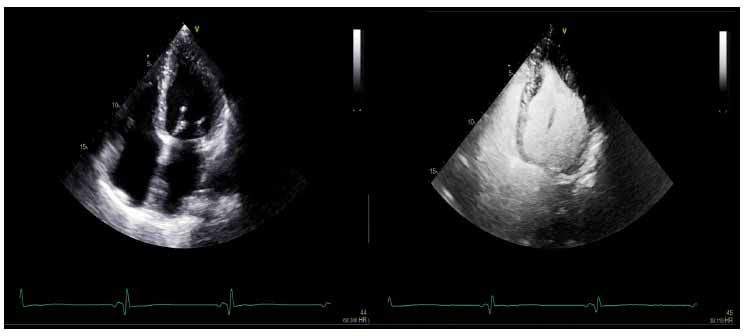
Apical 4 chamber TTE view without (left) and with (right) contrast enhancement, providing a better definition to asymmetric apical hypertrophy in a patient with apical HCM.
